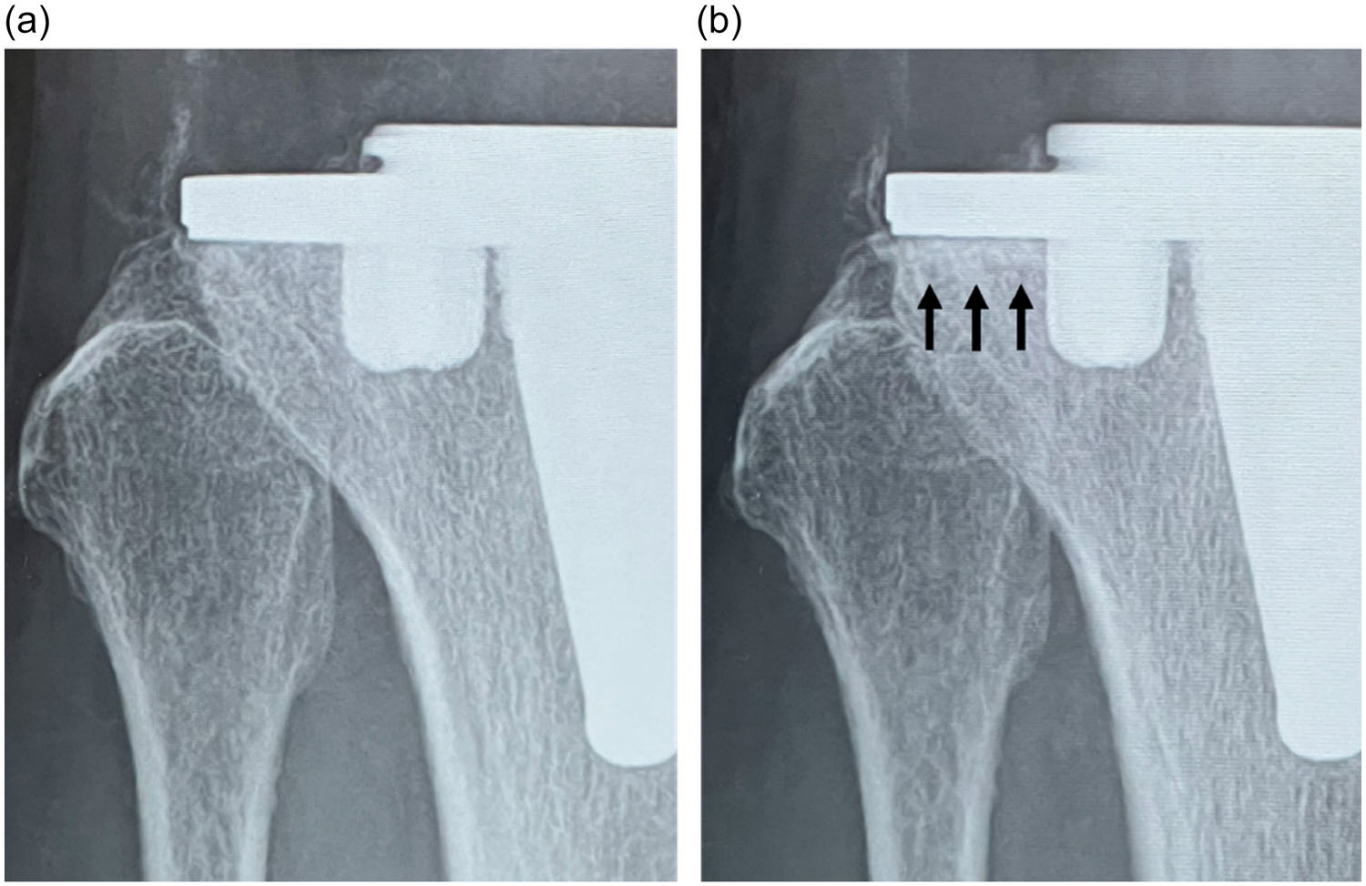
(a) Anteroposterior (AP) radiographs of a patient who underwent AFFIXIUM TKA at 1 week postoperatively. There was no bone on‐growth in Zone 2. (b) AP radiographs of a patient who underwent AFFIXIUM TKA at 2 months postoperatively. Bone on‐growth appeared in Zone 2 (arrow). TKA, total knee arthroplasty.
